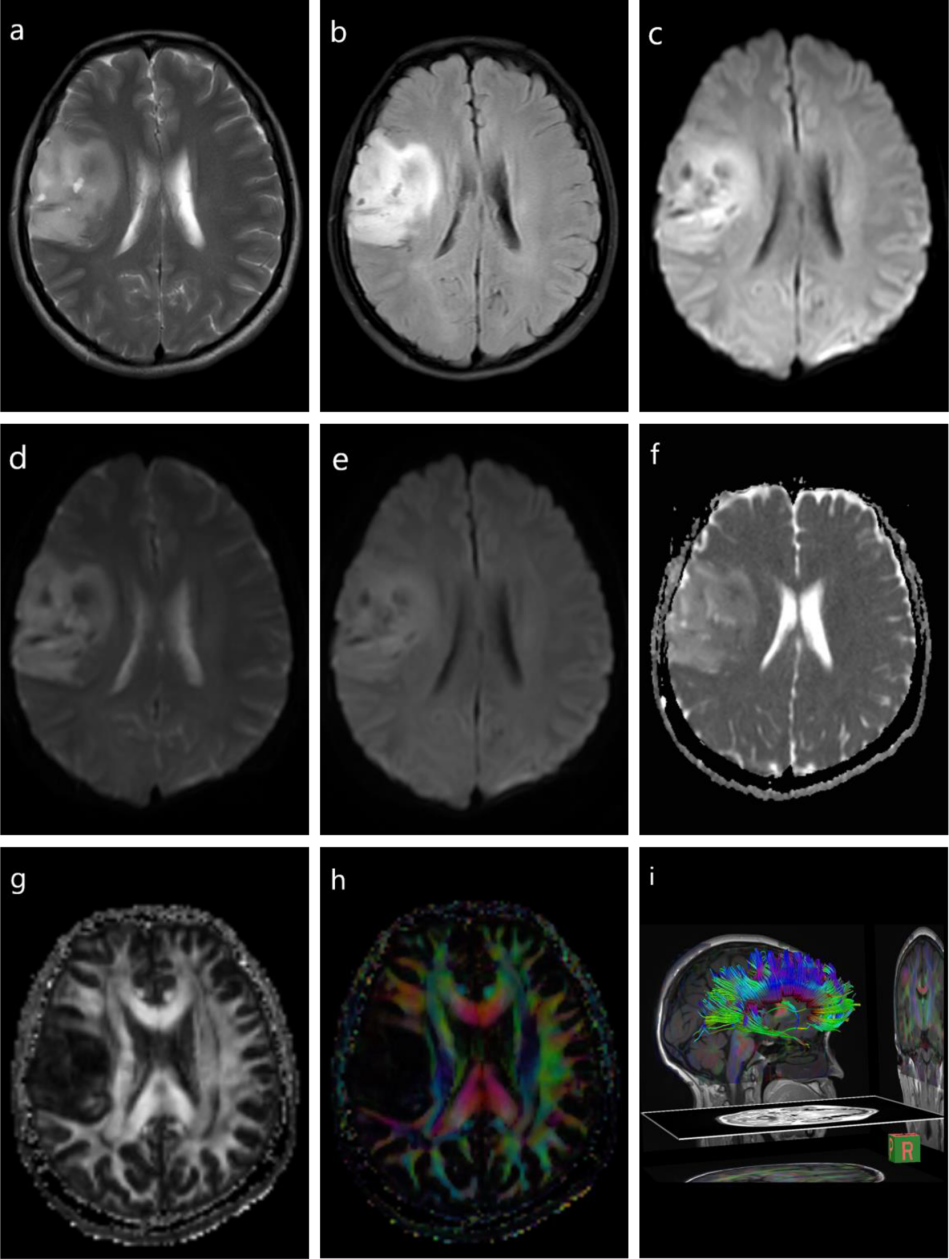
Diffusion-weighted imaging (DWI) and diffusion tensor imaging (DTI) of a glioma. T1-weighted imaging (T1WI) (A), fluid-attenuated inversion recovery (FLAIR) (B), DWI (C), DWI with b = 0 s/mm^2^
